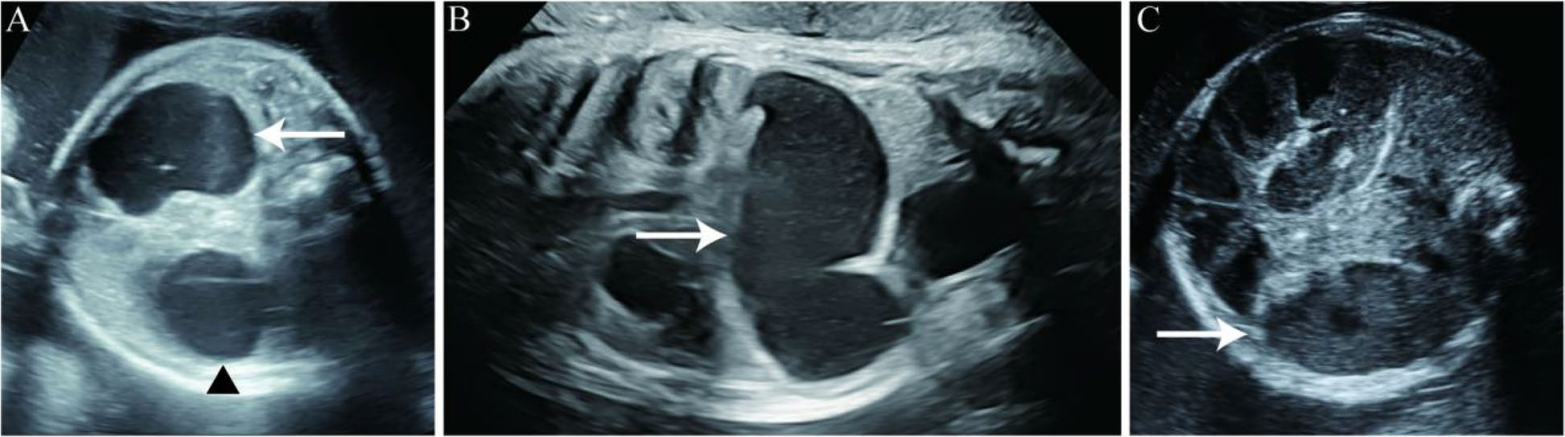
Representative prenatal ultrasound images from cases in the study. A Prenatal sonographic evaluation demonstrated a typical fetal double-bubble signal (black triangle: stomach, arrow: duodenum); B Coronal plane of intestinal dilatation (arrow: dilated bowel); C Cross section of intestinal dilation (arrow: dilated bowel)
